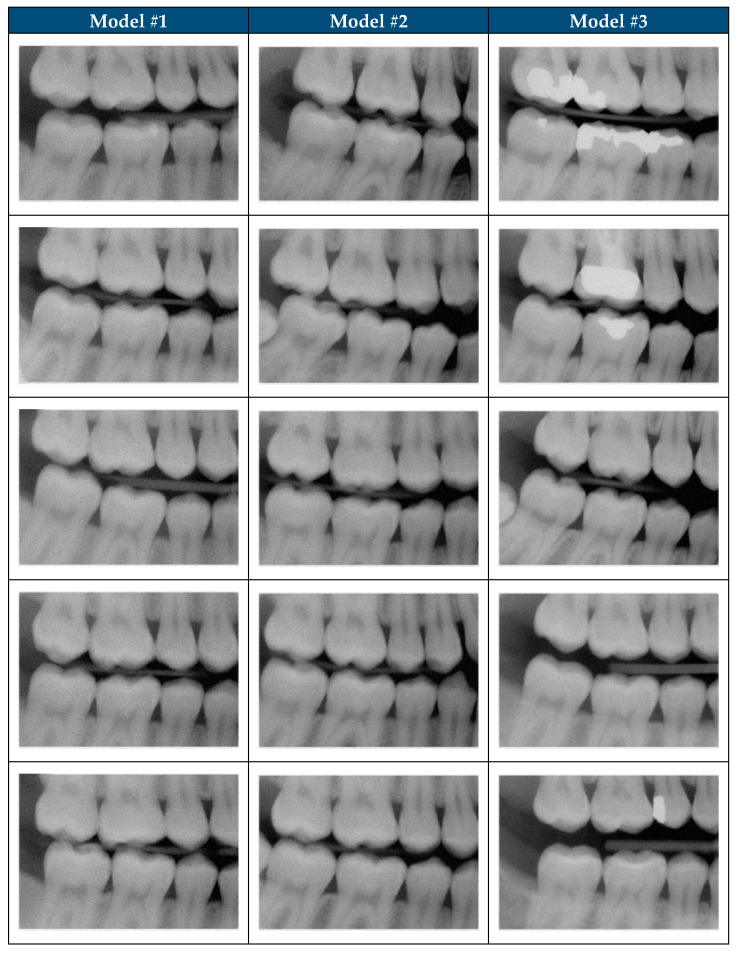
Realistic synthetic dental radiographs generated by the three diffusion models.
